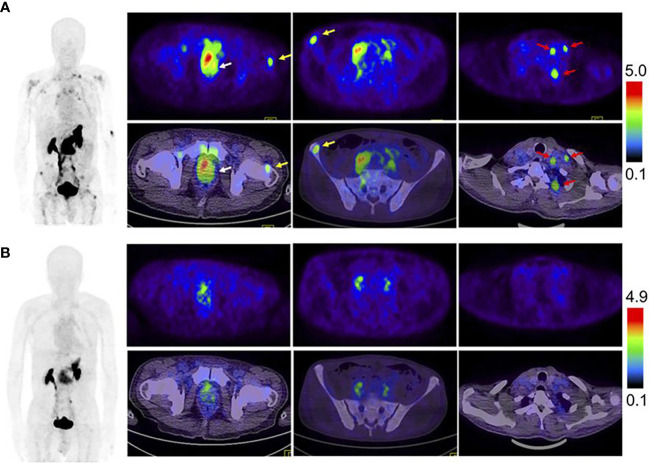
Comparison of ^68^Ga-RM26 PET/CT (A), and ^68^Ga-BBN PET/CT (B) in a 73-y-old man diagnosed as having PCa (white arrow) with lymph node involvement (red arrow) and bone metastasis (yellow arrow) before prostatectomy. ^68^Ga-RM26 PET/CT detected primary tumors, multiple lymph node involvement, and bone metastasis lesion, whereas those lesions showed much lower uptake on ^68^Ga-BBN PET/CT. Reprinted with permission from Zhang et al. (70). Copyright ^©^ 2018 by the Society of Nuclear Medicine and Molecular Imaging.
